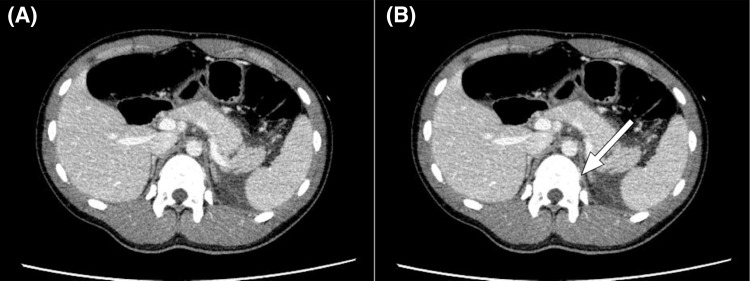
Abdominal CT scan of the patient showing a distal pancreatic transection with a small, peripancreatic fluid collection (American Association for Surgery of Trauma grade III) (A). CT found compression at the site of the injury corresponding to the area of compression between the vertebral body and the examiner’s hand during Ihara’s maneuver, as indicated by the arrow (B)
